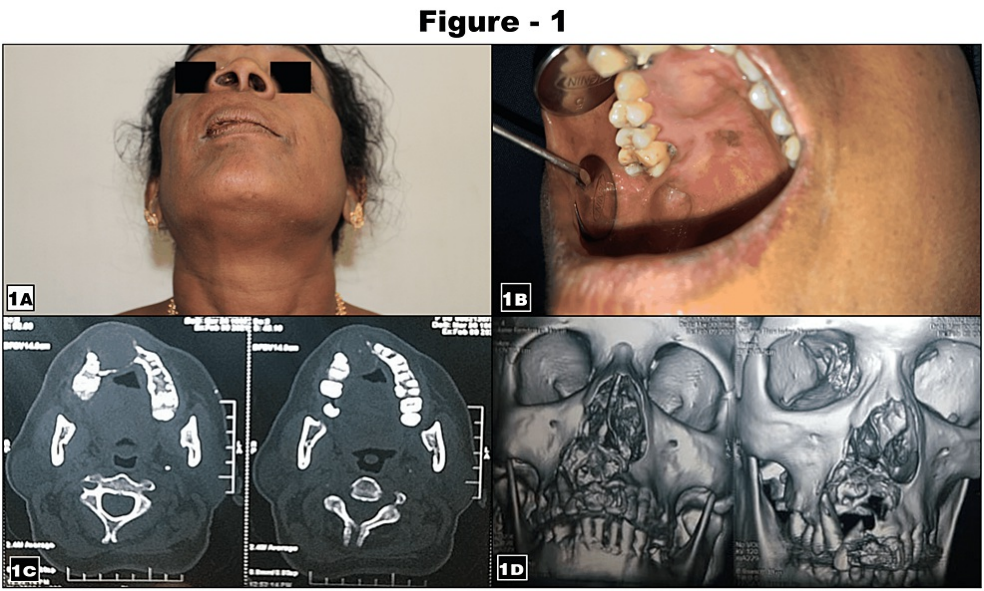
Preoperative clinical presentation, computerized tomography (CT), and three-dimensional imaging of recurrent pleomorphic adenoma 1A. Shows facial asymmetry in the upper anterior region, 1B. Shows intra-oral swelling in the anterior maxilla, 1C. CT imaging shows an osteolytic lesion evident on the right maxilla, and 1D. 3D imaging shows a destructive lesion
